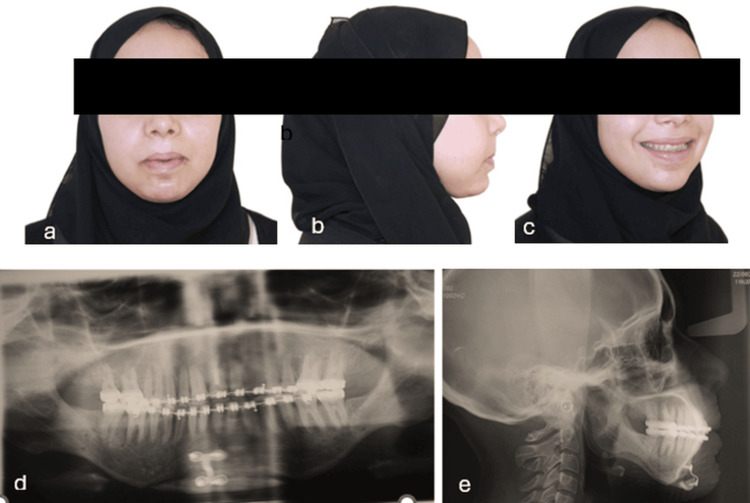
Postoperative assessment following advancement genioplasty and osteosynthesis plate removal. (a) Frontal extraoral photograph. (b) Right profile extraoral photograph. (c) Smiling extraoral photograph. (d) Panoramic radiograph obtained after plate removal. (e) Lateral cephalometric radiograph showing the skeletal and soft tissue changes achieved following advancement genioplasty.
